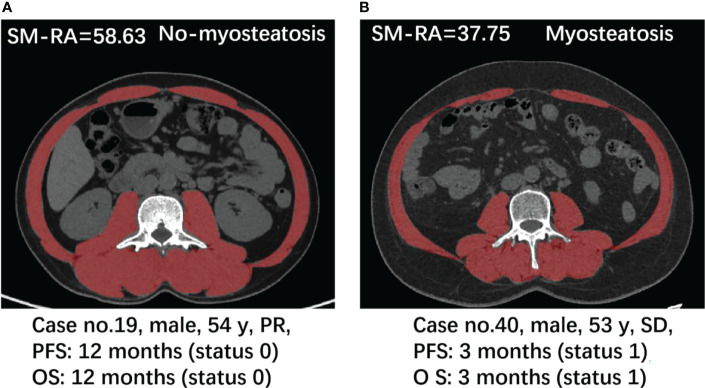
Case presentation. Axial CT images at the L3 level used for the measurement of multiple parameters for body composition, respectively in a 54-year-old man with PR (A) and a 53-year-old man with SD (B).
